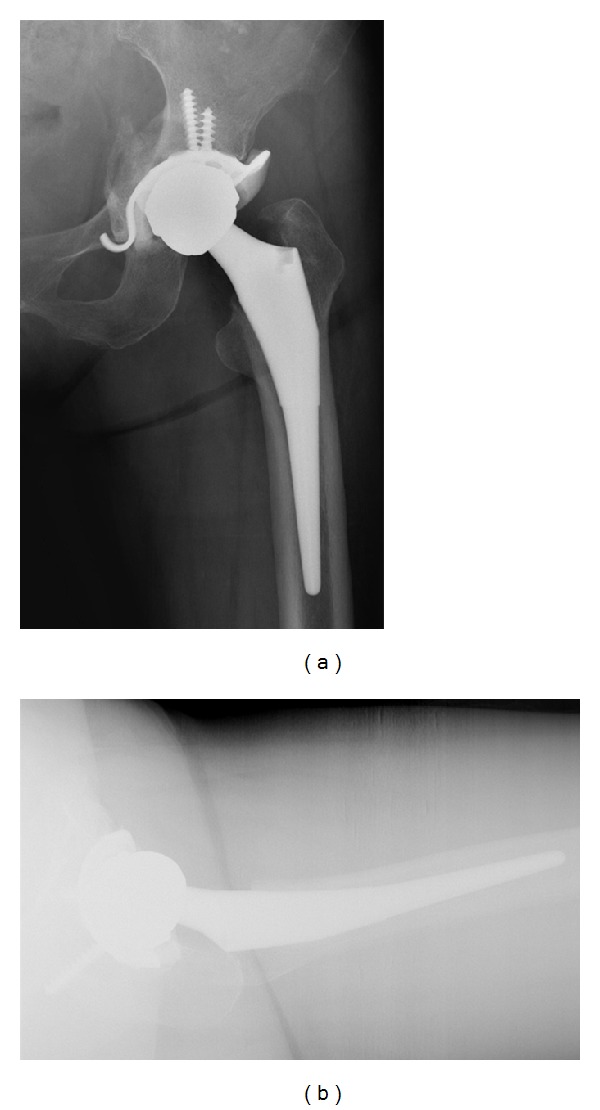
Radiographs taken 1 year after total hip arthroplasty showing well-fixed cementless femoral stem and consolidated medial acetabular bone graft with well-fixed acetabular cup ((a); (b)).
